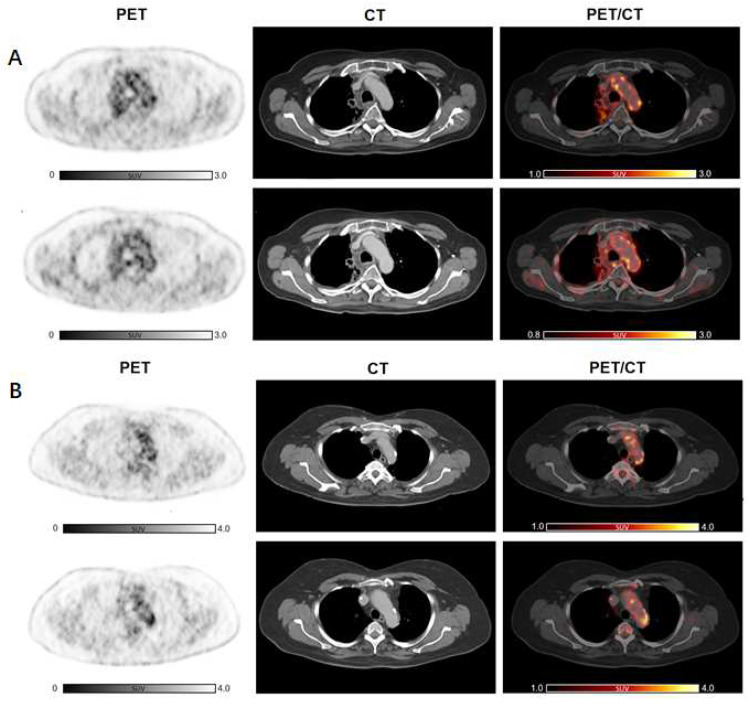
2-[^18^F]FDG arterial metabolic activity after ICI therapy compared to baseline in patients previously treated with other anti-cancer therapies. No significant increase in arterial 2-[^18^F]FDG uptake after immunotherapy can be detected in a subject already treated with RT (A) as well as in a patient who earlier underwent CHT and RT (B). Abbreviations: CHT, chemotherapy; 2-[^18^F]FDG, 2-[^18^F]fluoro-d-glucose; ICI, immune checkpoint inhibitors; RT, radiotherapy.
